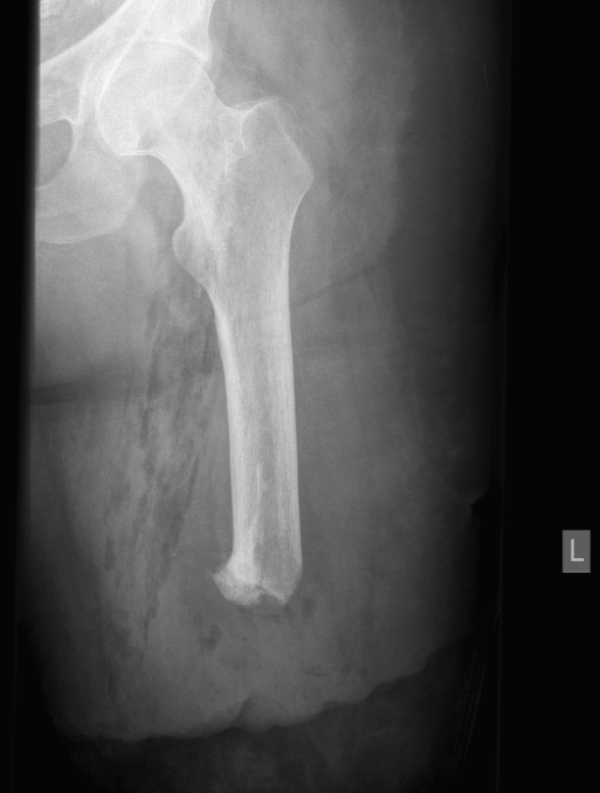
Roentgenogram upper leg after removal of calcifications.
